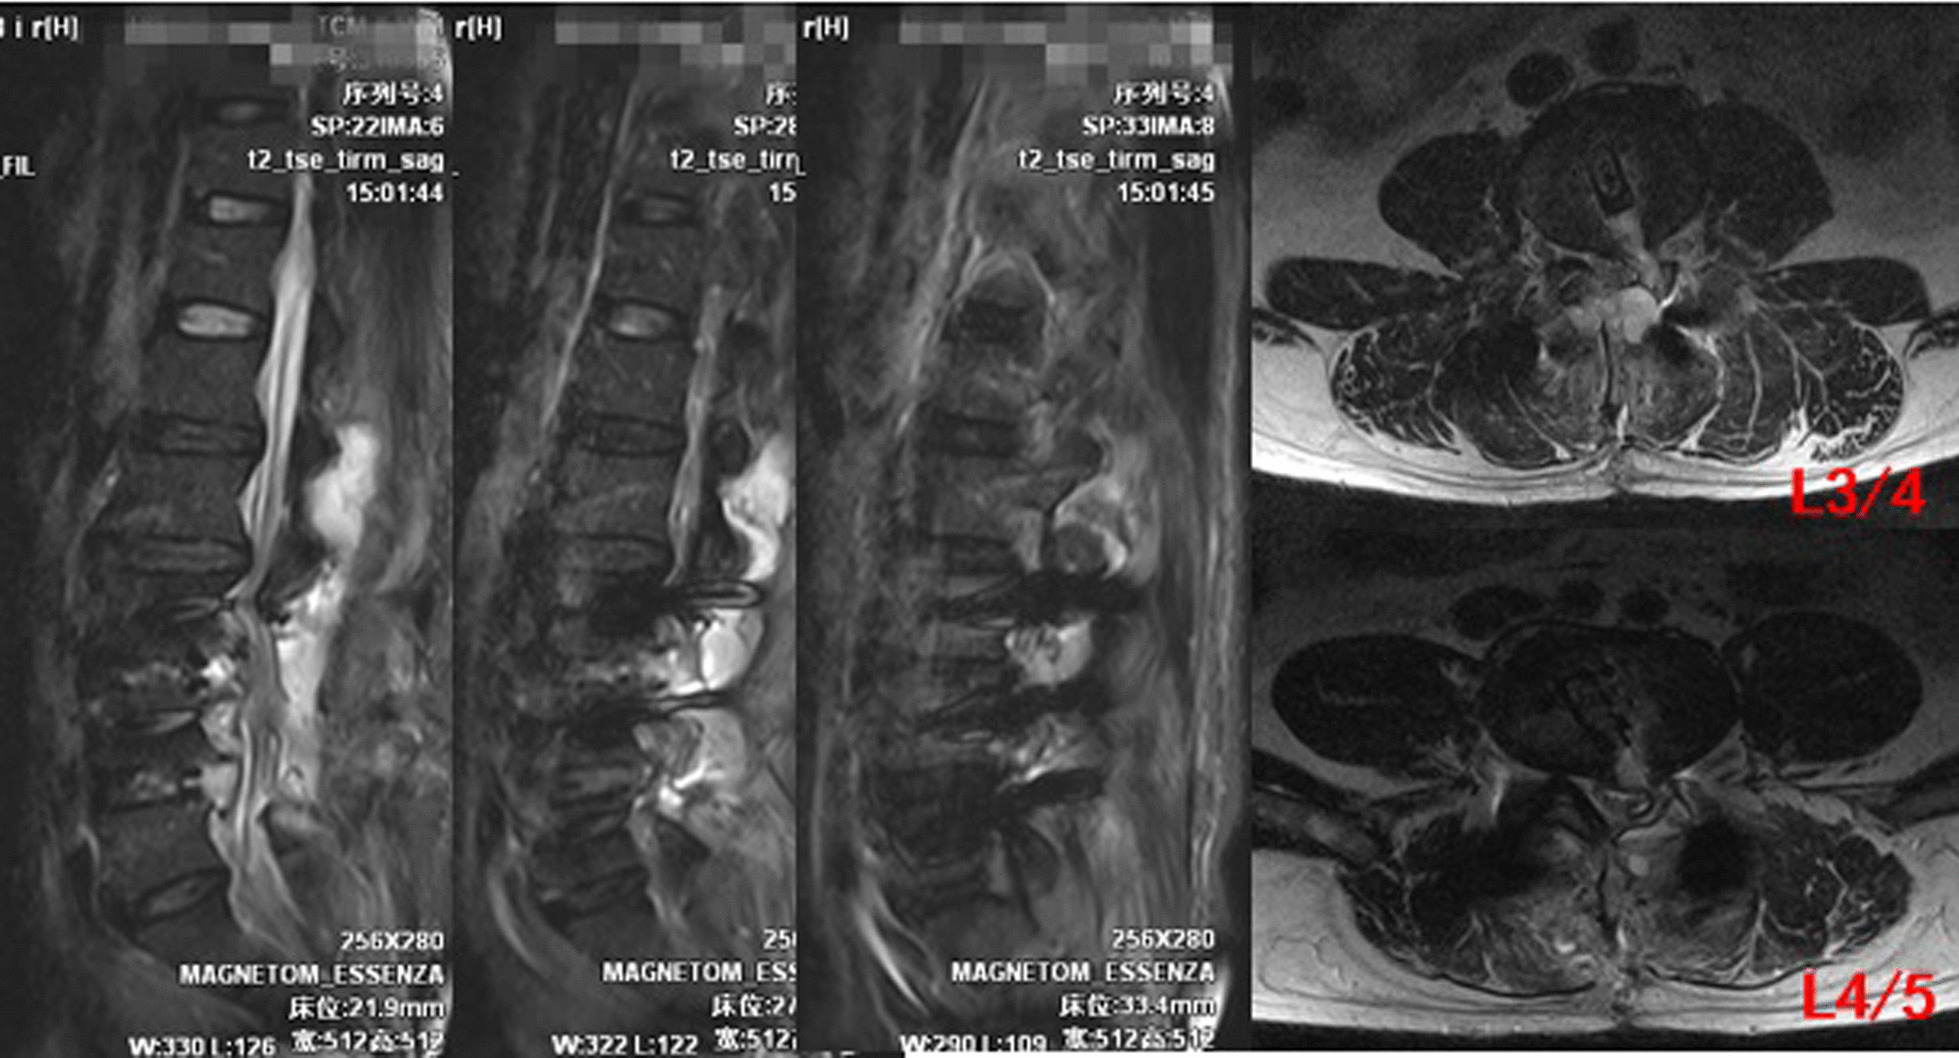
MRI was reexamined on the 12th day after the operation
